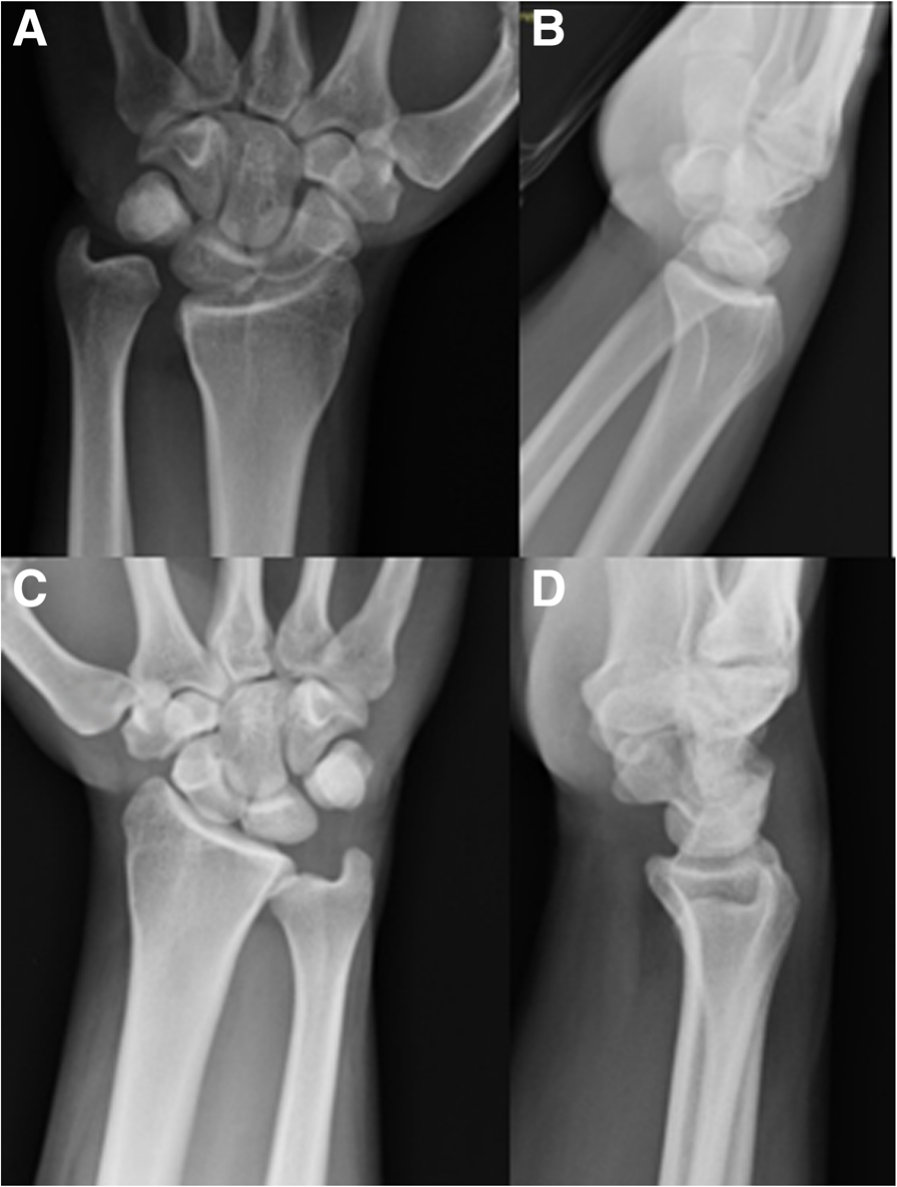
Clinical case 4, pre-operative: wrist. Pre-operative X-rays of the same patient. The left wrist (a, b) highlighted a DRUJ lesion, more evident if compared to the right unaffected wrist images (c, d)
